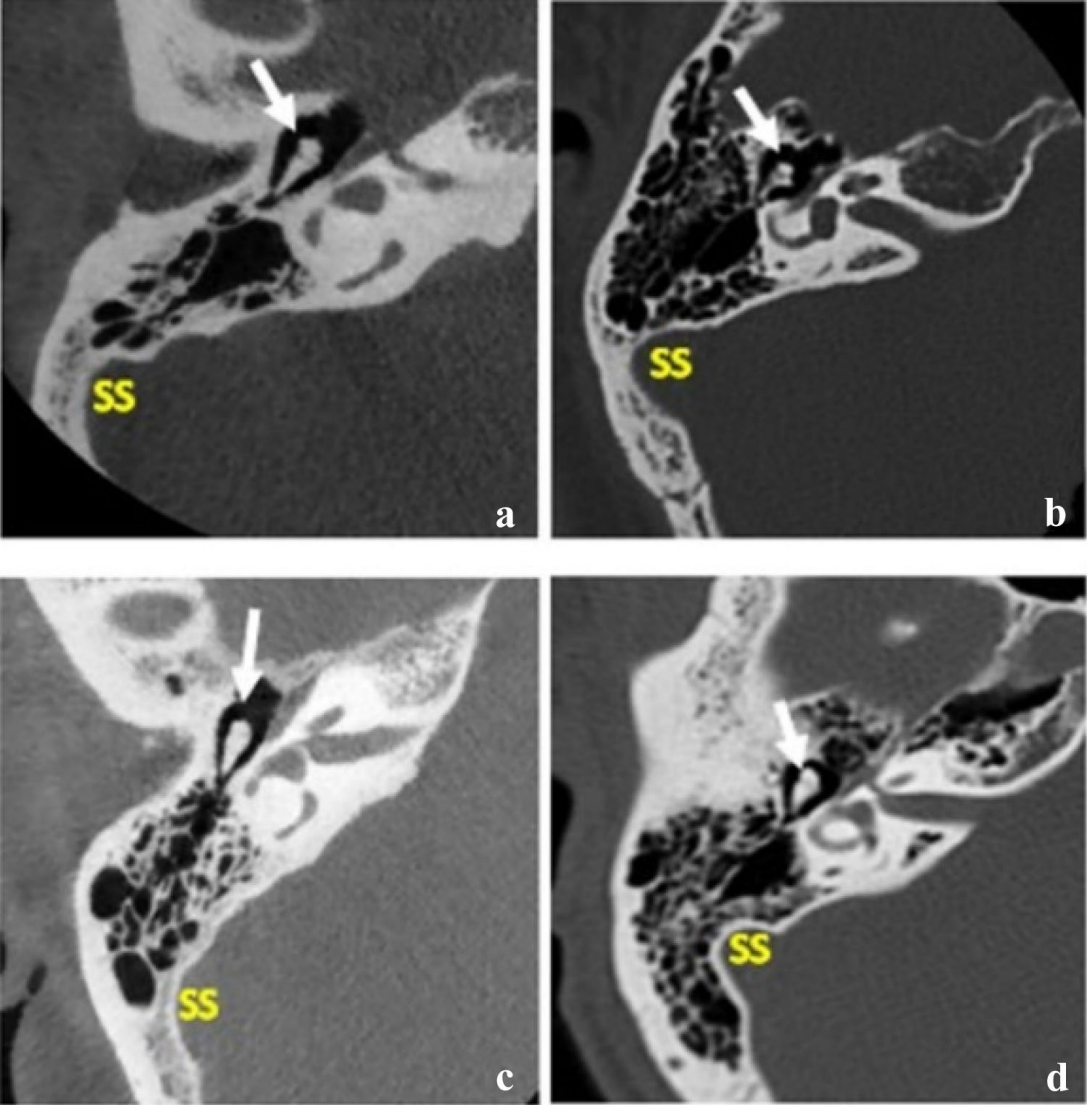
A representation of axial CT images taken at the level of the MIJ (white arrow) used in the questionnaire showing different degrees of pneumatization after removing the arbitrary lines. a hypo-pneumatization; b moderate pneumatization; c good pneumatization; d hyper-pneumatization. *SS* sigmoid sinus (Color figure online)
